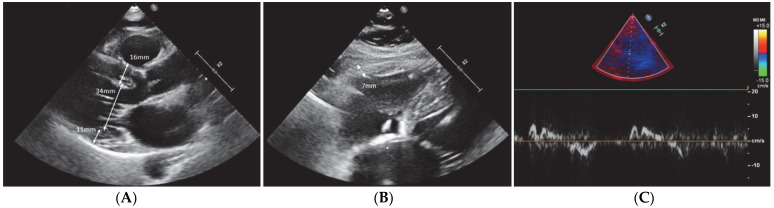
Transthoracic 2D echocardiography of the grandmother: (A) Parasternal long axis view showing asymmetrical LV hypertrophy (interventricular septum 16 mm; posterior wall 11 mm); (B) subcostal view focused on the right ventricle indicating mild RV hypertrophy (RV free wall thickness of 7 mm); (C) pulsed-wave tissue doppler (septal mitral annulus site) showing reduced myocardial velocities: s’ = 5.5 cm/s, and e’ = 6 cm/s.
